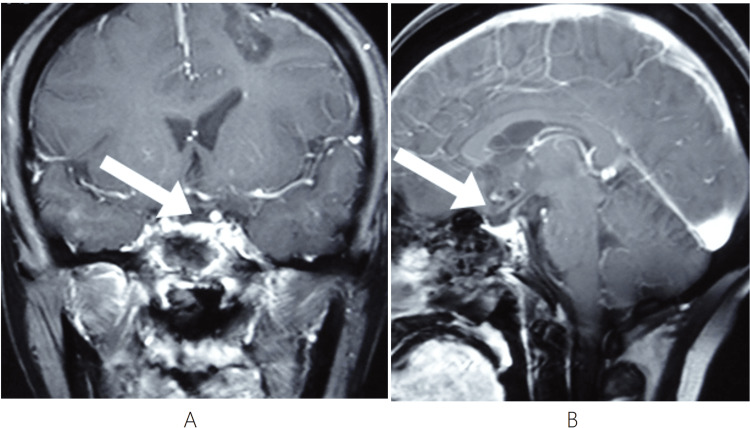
Post-surgery - March 2014 Definite residual disease in the sellar region post-surgery. (A) Coronal T1-weighted post-contrast image shows linear enhancement in the post-surgical sellar area (arrow). (B) Sagittal T1-weighted post-contrast image shows linear enhancement in the post-surgical sellar area (arrow).
